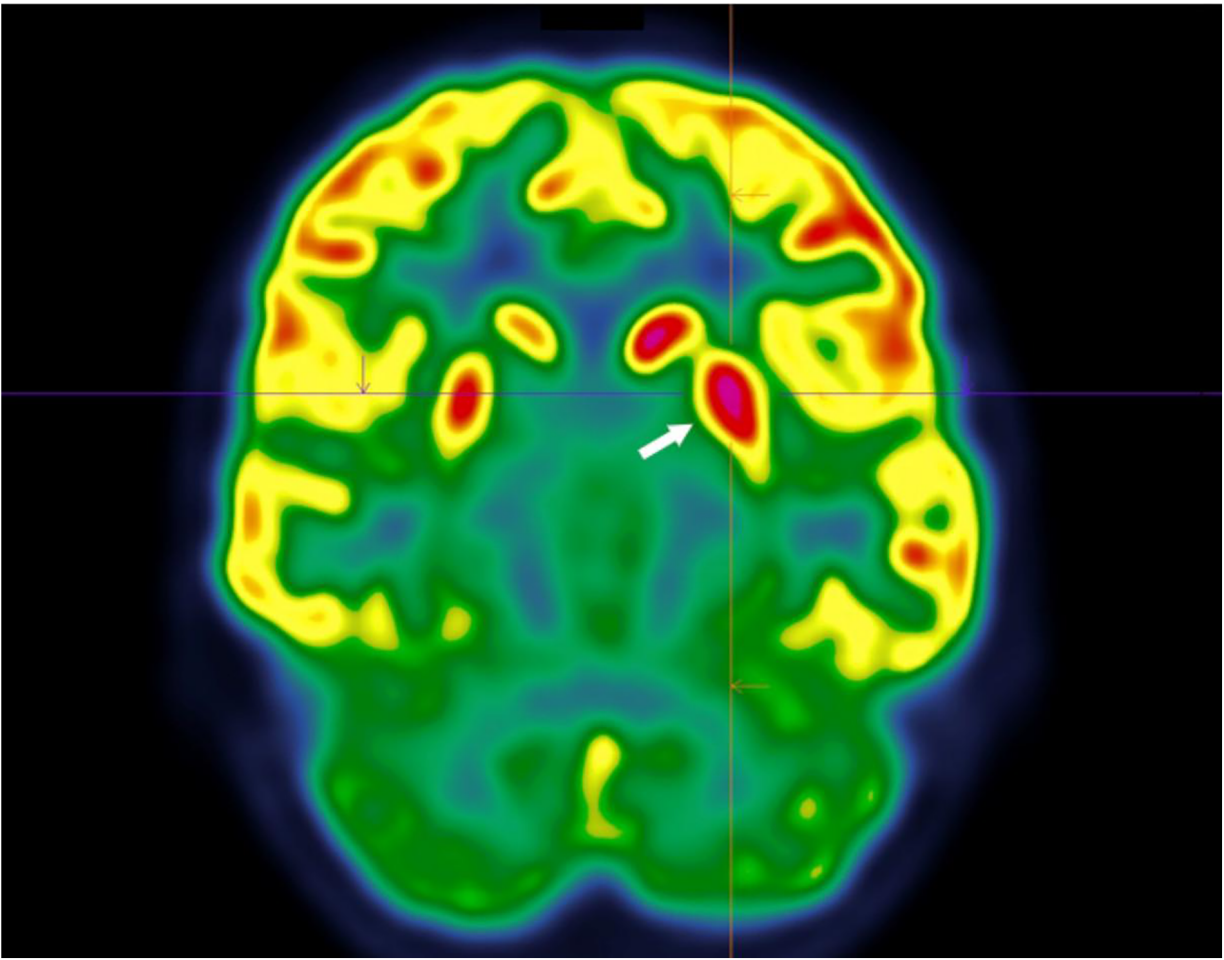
18F-FDG PET scan focused on the basal ganglia region showing asymmetry of the caudate nuclei and putamen, with predominant fixation on the left.
